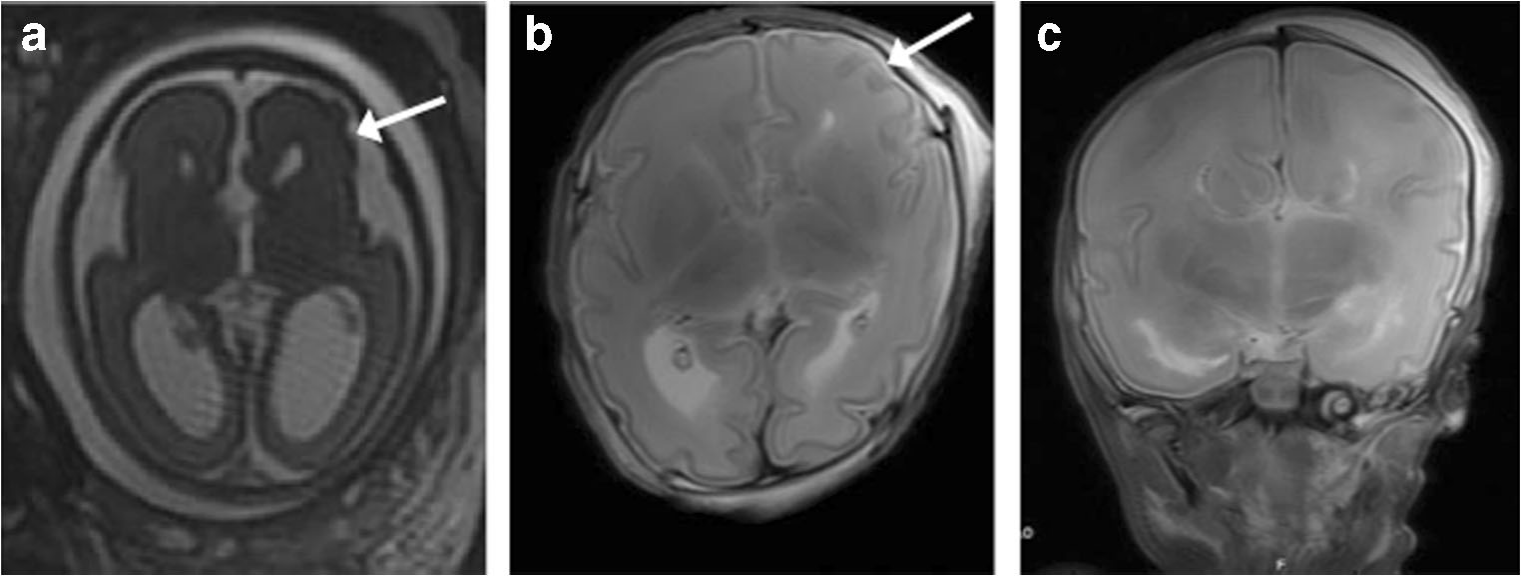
Agenesis of the corpus callosum (ACC) with unilateral left frontal oversulcation in a 29-week gestation fetus. a Axial T2-weighted imaging of the foetal brain on iuMR demonstrates the lack of callosal tissue and oversulcation in the left frontal lobe (white arrow). b Axial T2-weighted PMMR in the same fetus shows similar results, although the oversulcation (white arrow) is less marked. c Coronal T2-weighted PMMR of the fetus shows the typical steer-horn appearance in absent corpus callosum
